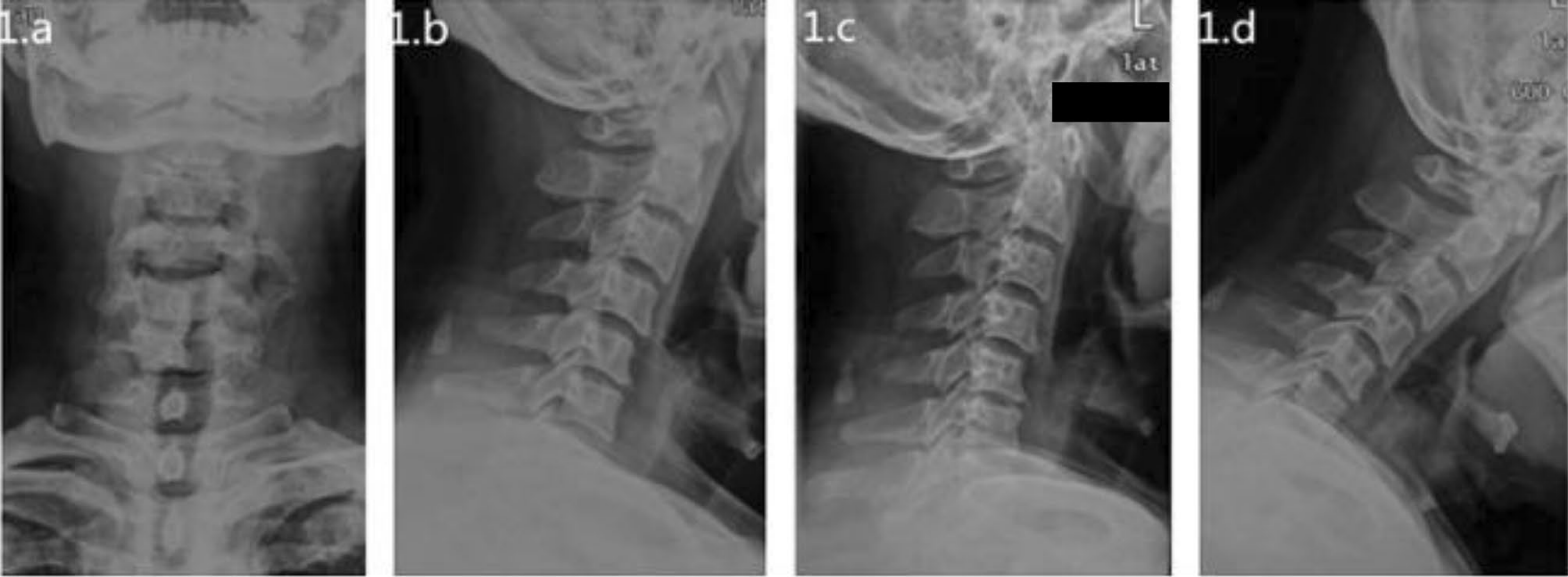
Preoperative cervical X-ray. The anteroposterior and lateral images of the cervical vertebra (a) and (b) showed degeneration of the cervical vertebra, and the physiological curvature was straightened. Cervical vertebral hyperextension and flexion images (c and d) showed no cervical vertebral stability.
